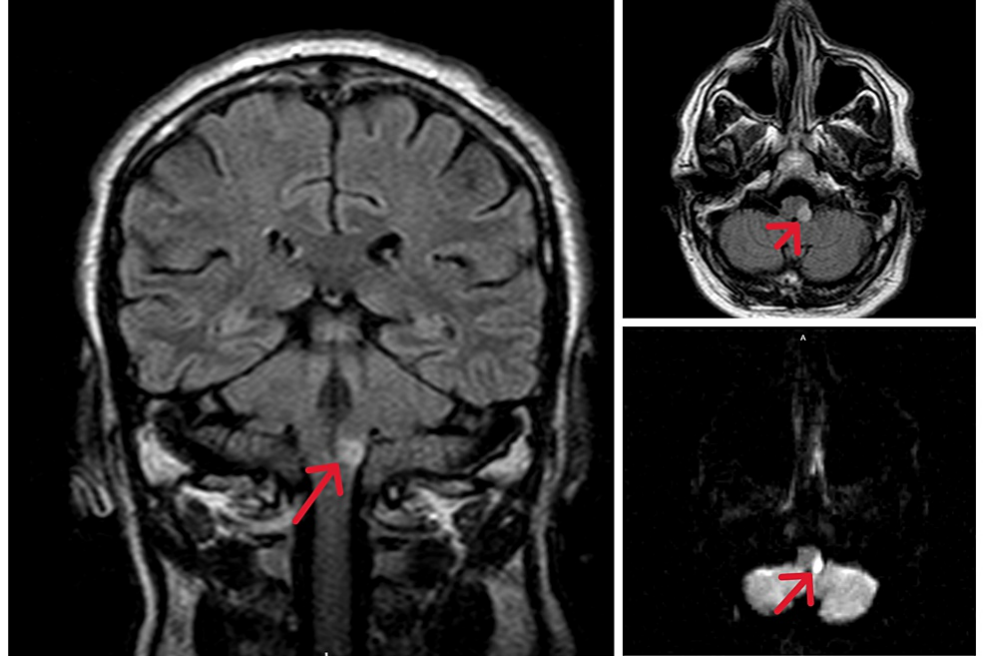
MRI of the brain FLAIR imaging (first picture: coronal and left upper axial) showing restricted diffusion in the left superior posterolateral medulla (red arrow) consistent with ischemia associated with DWI (lower left) and high signal in this region with mass effect, suggestive of posterior inferior cerebellar artery infarct. MRI: magnetic resonance imaging; FLAIR: fluid-attenuated inversion recovery; DWI: diffusion-weighted imaging
